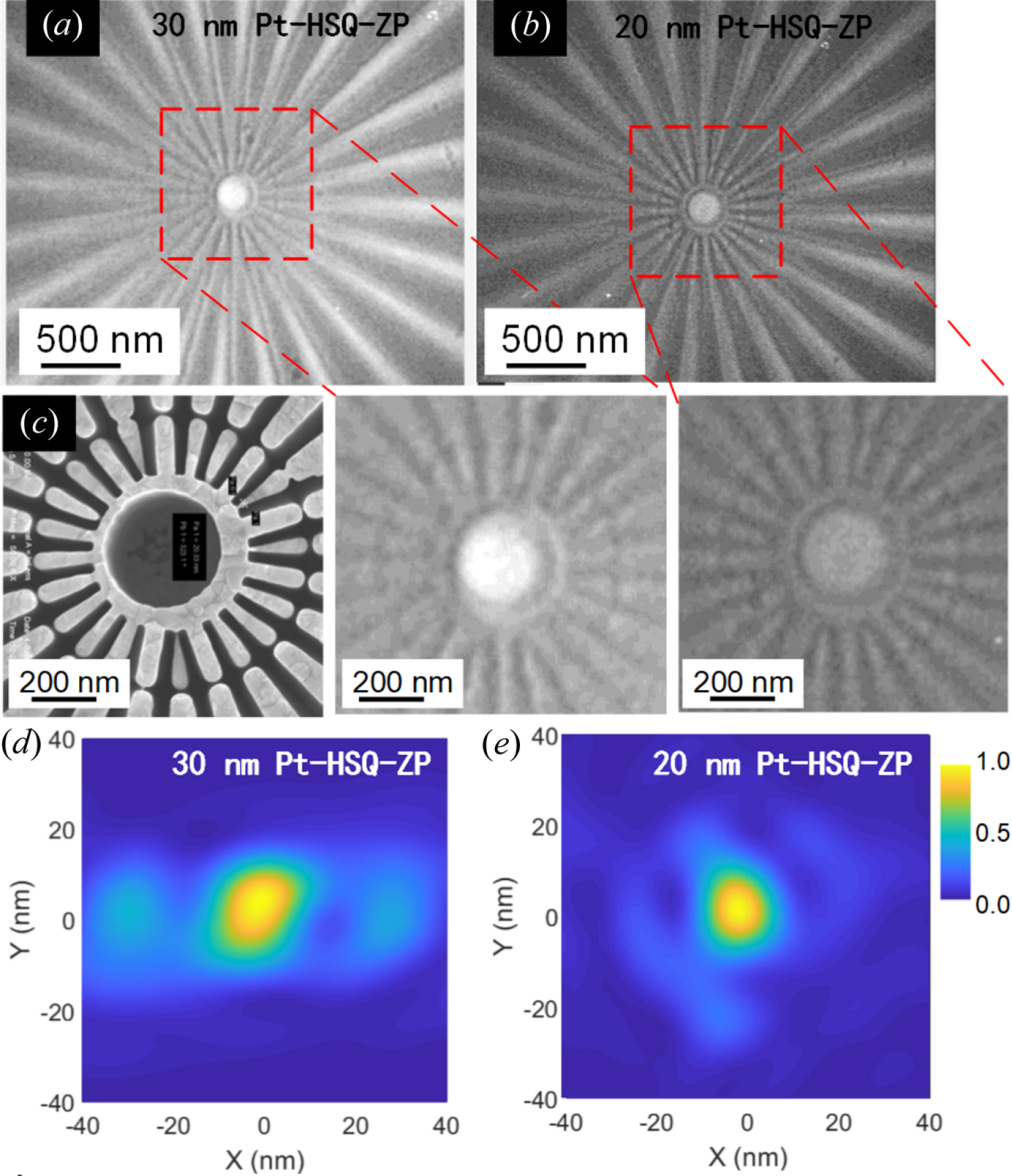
Focusing and imaging quality of the Pt-HSQ-ZPs measured using TXM. TXM images of a Siemens star test sample using the Pt-HSQ-ZPs with resolutions of (*a*) 30 nm and (*b*) 20 nm. The energy was 7.2 keV and the dwell time was 10 s. (*c*) SEM image of the Siemens star with a minimum resolution of 20 nm. Focusing performance characterization using X-ray ptychographic CDI and 2D intensity distribution for (*d*) 30 nm and (*e*) 20 nm Pt-HSQ-ZPs.
